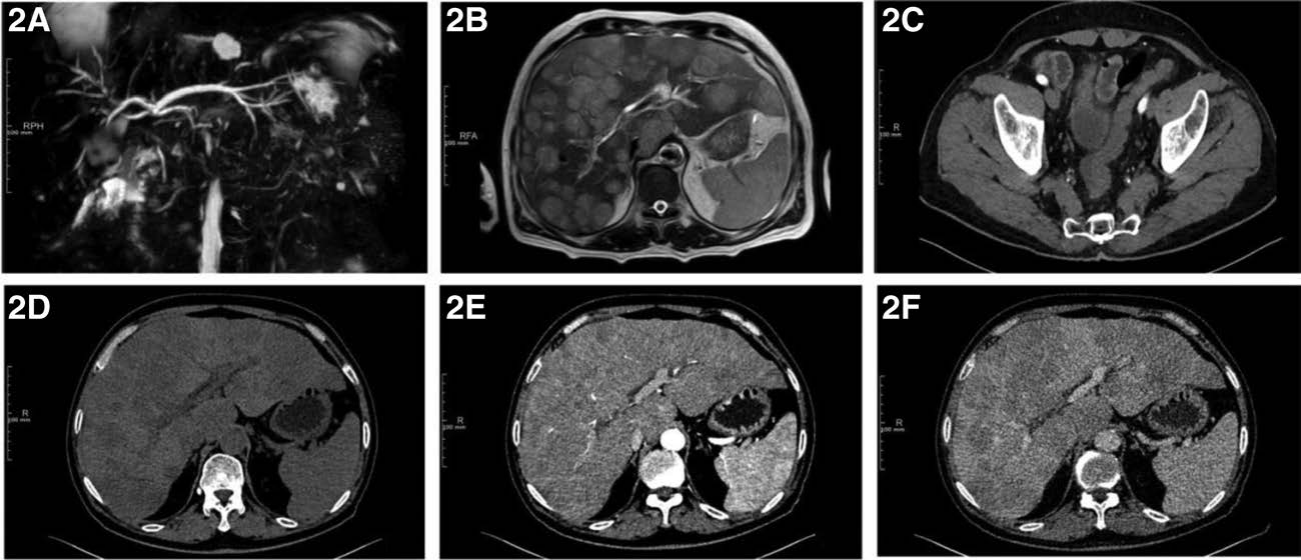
(A) MRCP showed that the common hepatic duct and the upper part of the common bile duct are not clearly visible, and the end of the common bile duct is faintly visible. (B) MRCP also showed the diffuse heterogeneous lamellar slightly long-long T2 signal shadow in the liver. (C) Computerized tomography (CT) showed sigmoid colon is stiff with narrow lumen and lymph node shadow around it. (D–F) There were low and slightly low density on plain scan CT and progressive enhancement when enhanced in Liver. CT = computerized tomography, MRCP = magnetic resonance cholangiopancreatography.
